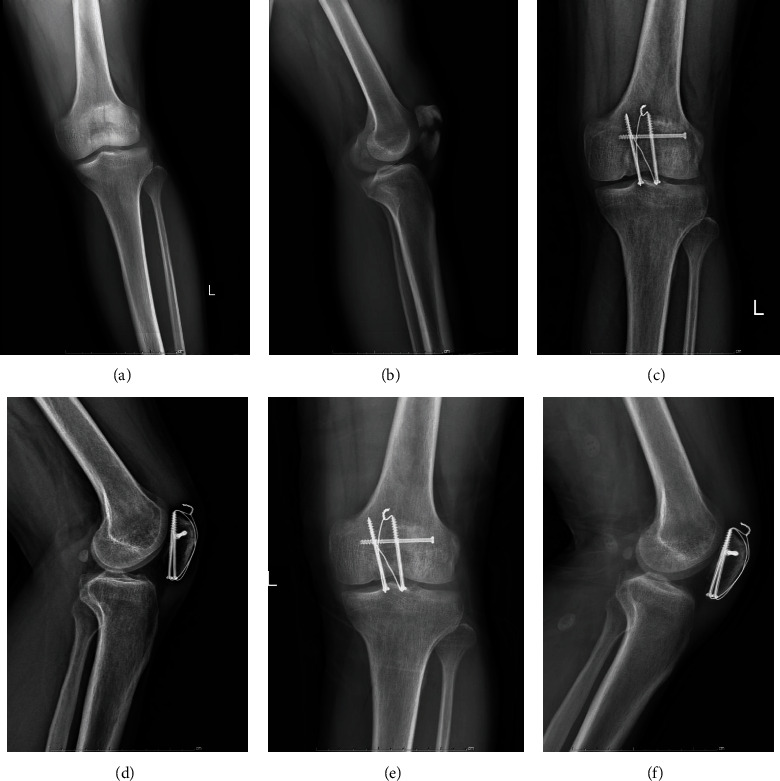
(a–f) A 37-year-old man with a transverse patellar fracture had cannulated screws tension band fixation. (a) An anteroposterior X-ray shows the transverse patellar fracture after injury. (b) A lateral X-ray after injury. (c) A postoperative anteroposterior X-ray of 1 month. (d) A postoperative lateral X-ray of 1 month. (e) A postoperative anteroposterior X-ray of 6 months. (f) A postoperative lateral X-ray of 6 months.
